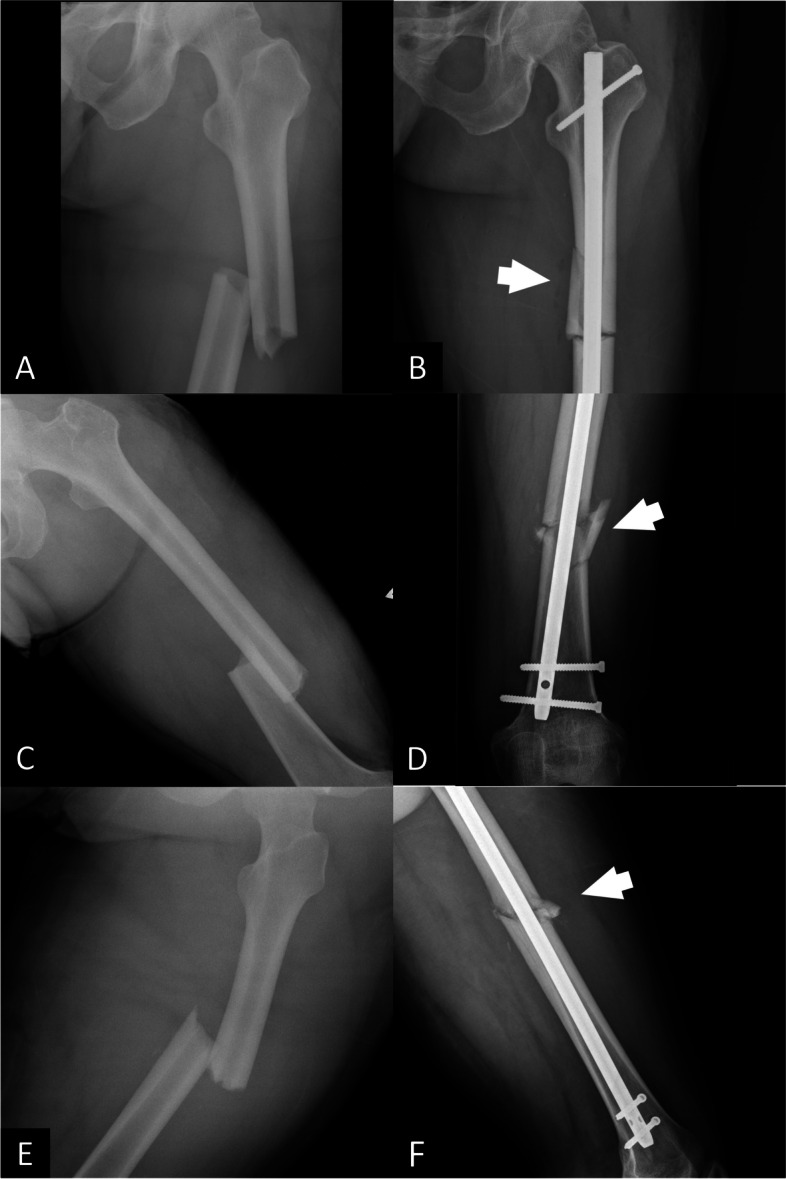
A A preoperative plain film of a transverse femoral shaft fracture, B a postoperative plain film showing an iatrogenic fracture comminution involving the medial cortex with proximal extension, C a preoperative plain film of a transverse femoral shaft fracture, D a postoperative plain film showing iatrogenic fracture comminution involving the lateral cortex with distal extension, E a preoperative plain film of a transverse femoral shaft fracture, F a postoperative plain film showing iatrogenic fracture comminution involving the anterior cortex with proximal extension
